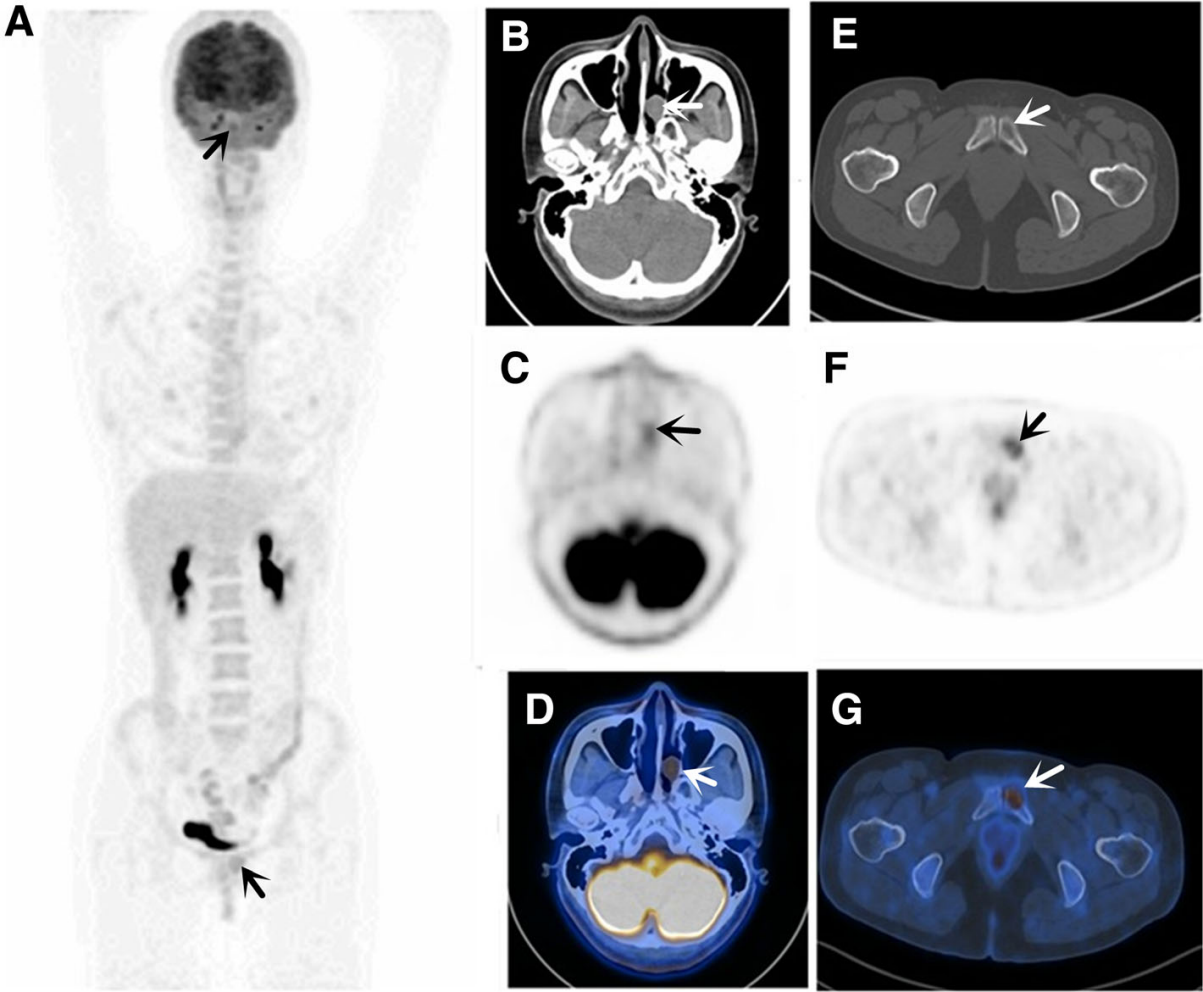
Case 8, a 36-year-old woman presented with nasal obstruction for three months, in whom the nasal endoscope showed the plasmacytoma in the left nasal cavity. Without evidence of systemic spread according to other clinical examination, suspicion of primary EMP of nasal cavity was made. ^18^F-FDG PET/CT was performed for further evaluation. CT (b), PET (c) and fused PET/CT (d) images showed ^18^F-FDG uptake in EMP of the nasal cavity. Another osteolytic lesion in the left pubis was identified by PET/CT (a, e-g). A biopsy of the pubis lesion was performed, which showed neoplastic-appearing plasma cells involved in the bone. Due to detection of new lesions in the bone, more invasive therapy was given, including enlarged field irradiation and systemic therapy, instead of resection of nasal plasmacytoma combined with RT alone
